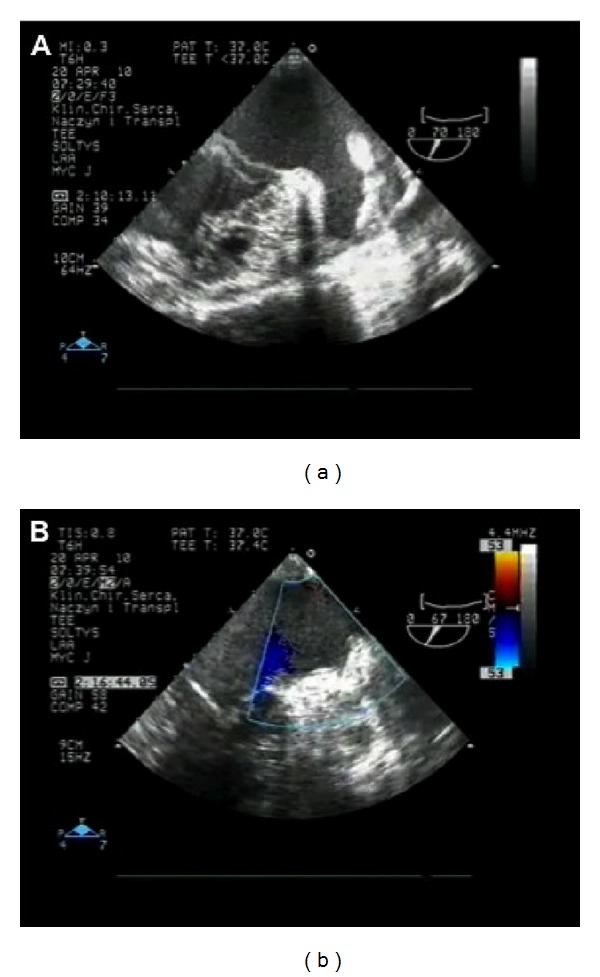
Transesophageal echocardiographic images of the left atrial appendage (a) prior to and (b) immediately after LARIAT suture deployment, highlighting acute LAA exclusion. (*Image courtesy of SentreHeart, Inc.*)
